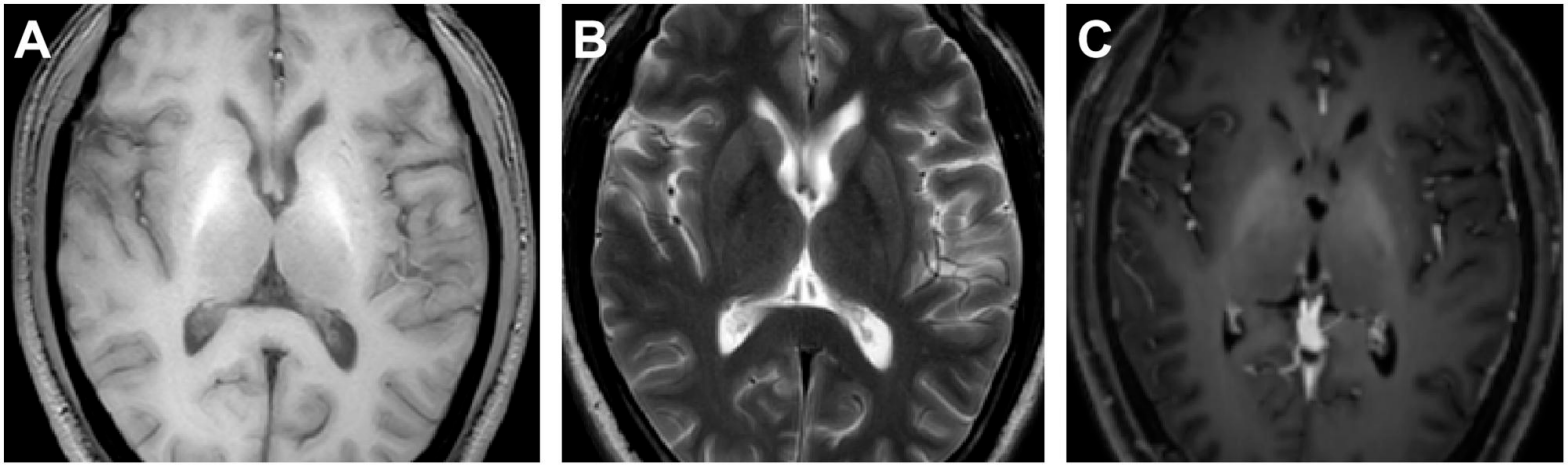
A 58-year-old male with hepatic encephalopathy. The patient presents with symmetrical abnormal signal regions in the bilateral globus pallidus. These regions demonstrate high signal intensity on T1-weighted magnetic resonance imaging (MRI) (A) and slightly low signal intensity on the corresponding T2-weighted MRI (B). Post-contrast T1-weighted MRI (C) reveals no significant enhancement.
